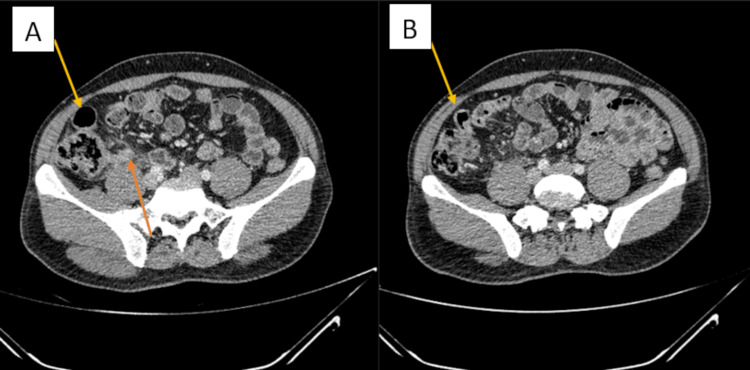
Pre-operative CT images show acute appendicitis; there were no findings that could indicate the congenital adhesions encountered on laparoscopy A: Terminal ileum (yellow arrow) is close to the abdominal wall but not shown to be adhered to it. Acute appendicitis is indicated by the orange arrow. B: Continuation of A, the adhered segment of the small bowel is the terminal ileum (yellow arrow) as it enters the cecum.
